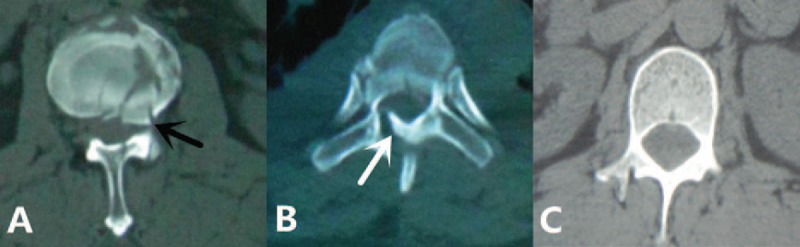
Different types of burst fractures in computed tomography scan: (A) asymmetric bone fragment intrusion into the spinal canal (the black arrow); (B) vertical lamina fracture (the white arrow); (C) normal vertebrae.
